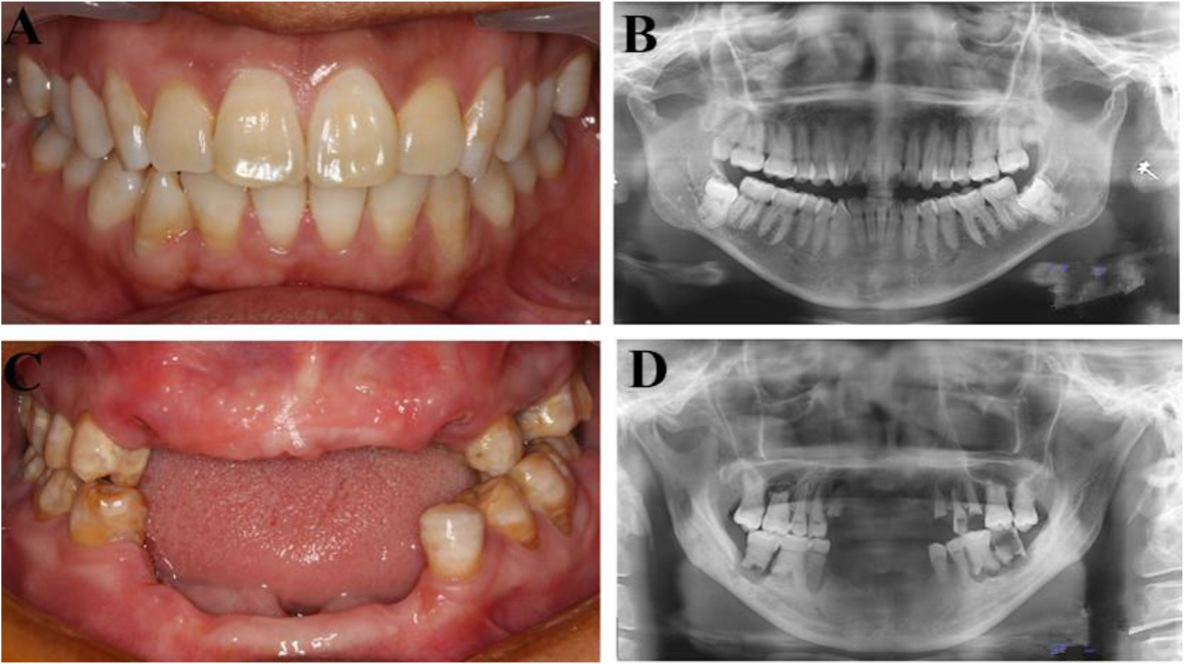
Intraoral images and panoramic radiographs of individuals. a Intraoral image of a normal subject. b Panoramic radiograph of normal subject. c Intraoral photo of a 47 year old subject affected by DD-. d Panoramic radiograph of a subject affected by DD-I
